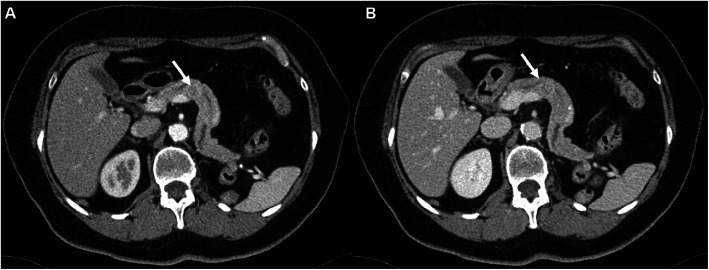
Axial CT images of the pancreas in arterial (A) and portal venous (B) phases of a 65-year-old woman with metastatic RCC. In both phases the metastasis is visible as a hypervascularized lesion (white arrows)
